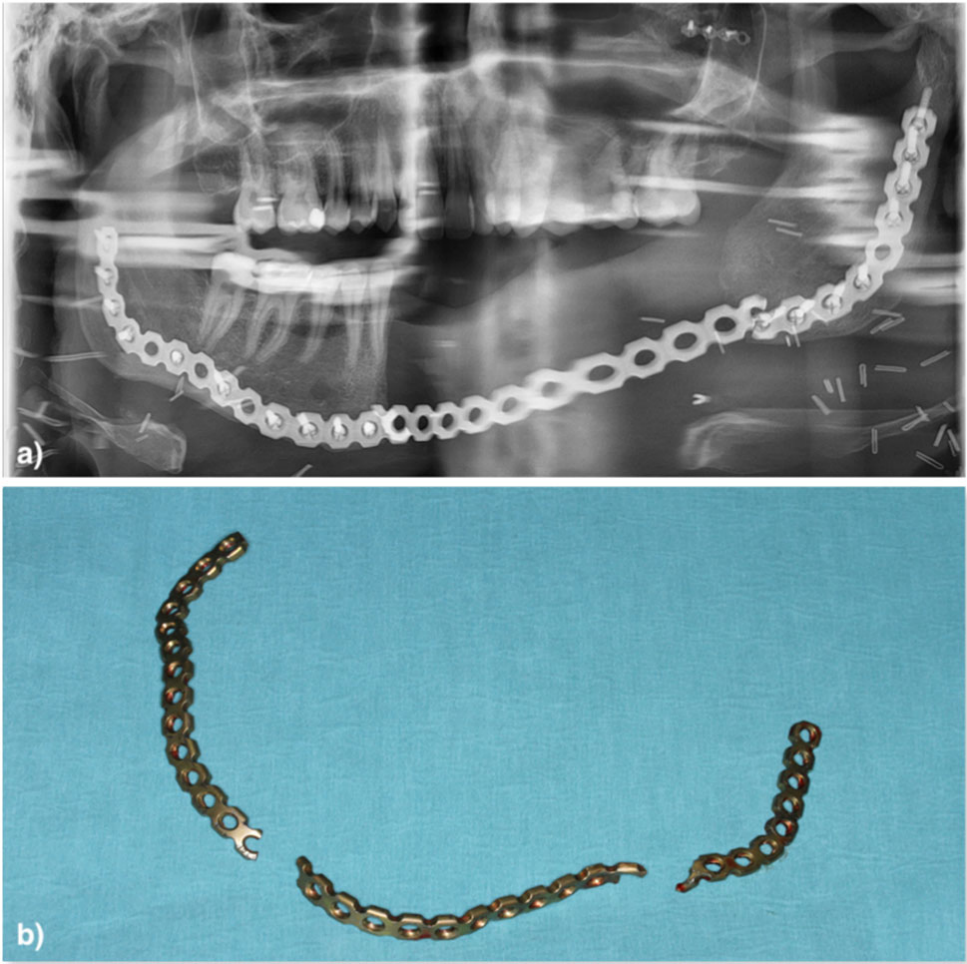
a Panoramic X-ray of a fractured reconstruction plate, b clinical view after fracture
